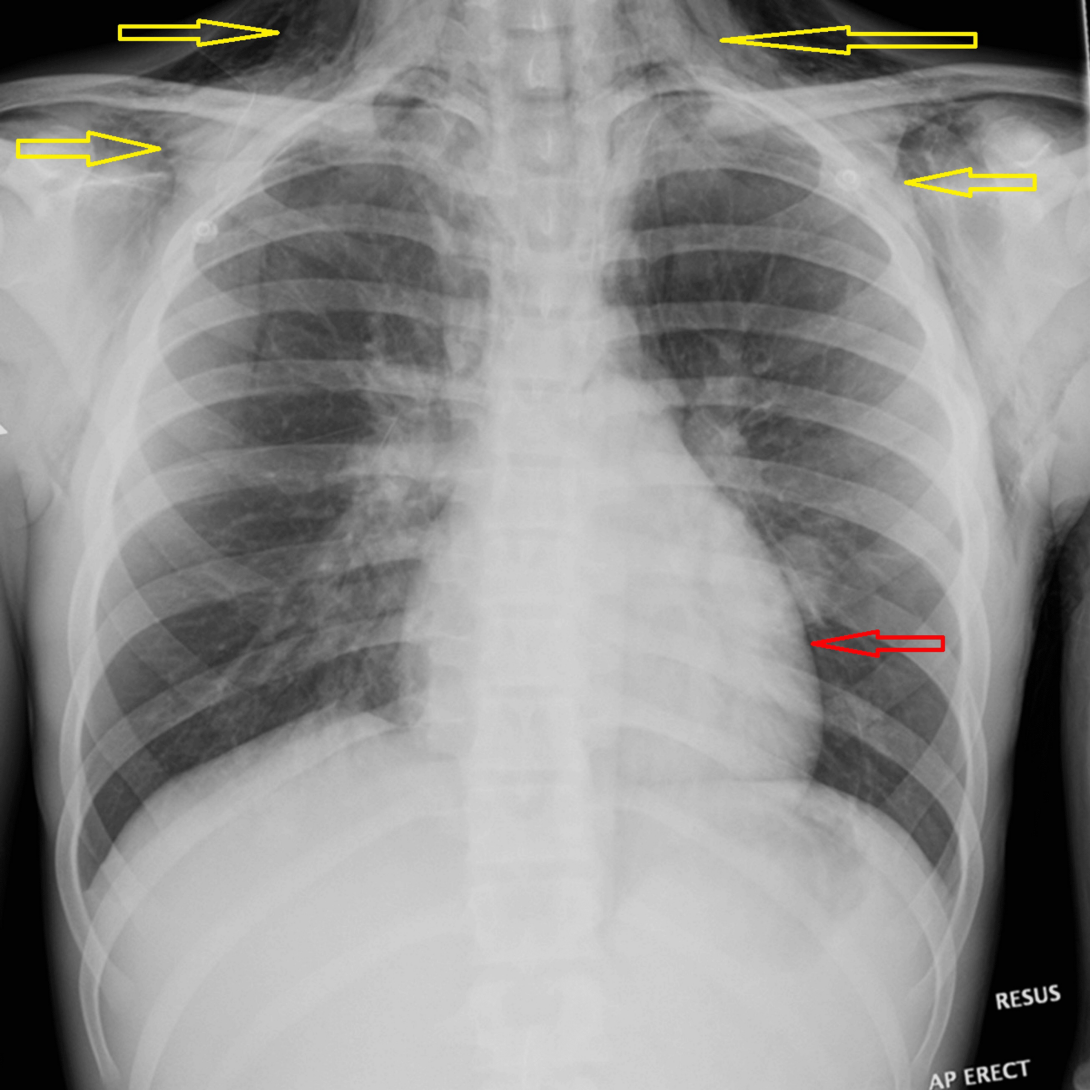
Chest X-ray AP erect chest radiograph showing diffuse subcutaneous emphysema (yellow arrows) tracking along the soft tissues of the neck and upper chest wall, with associated pneumomediastinum (red arrow).
